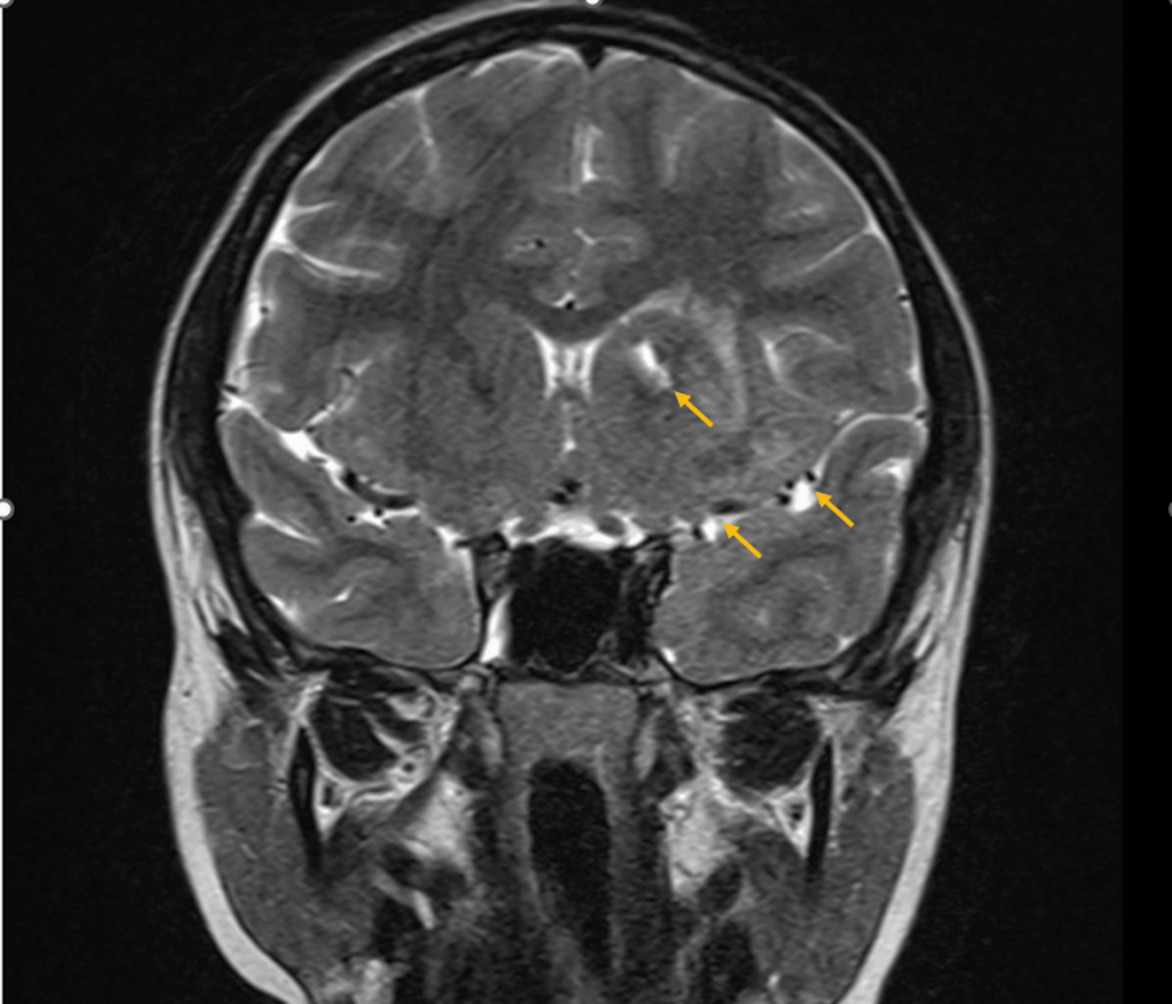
MRI of the brain showing ill-defined T2-weighted hyperintensities with tiny cystic spaces, multiple calcifications, and microhemorrhages involving the left gangliocapsular region, left parietooccipital, and left temporal cortex (yellow arrows).
